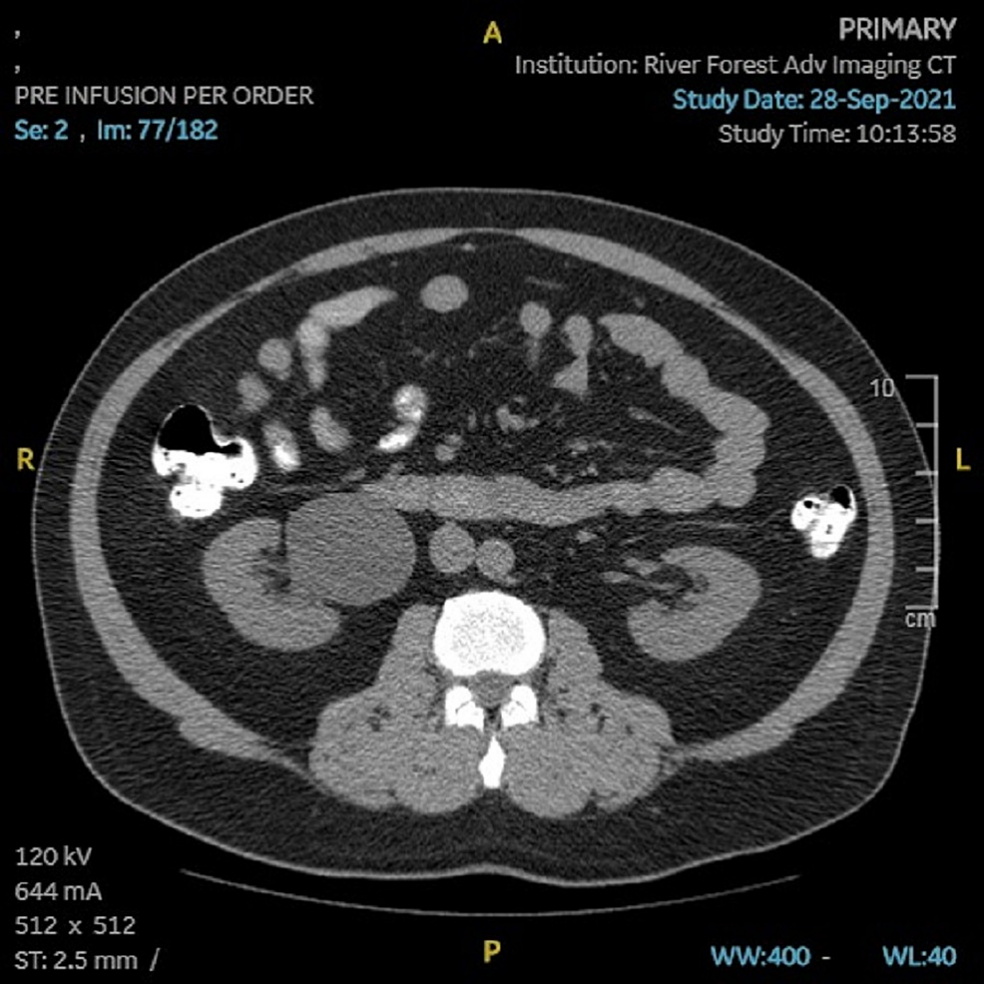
CT image (transverse) showing a very large right renal pelvis.
